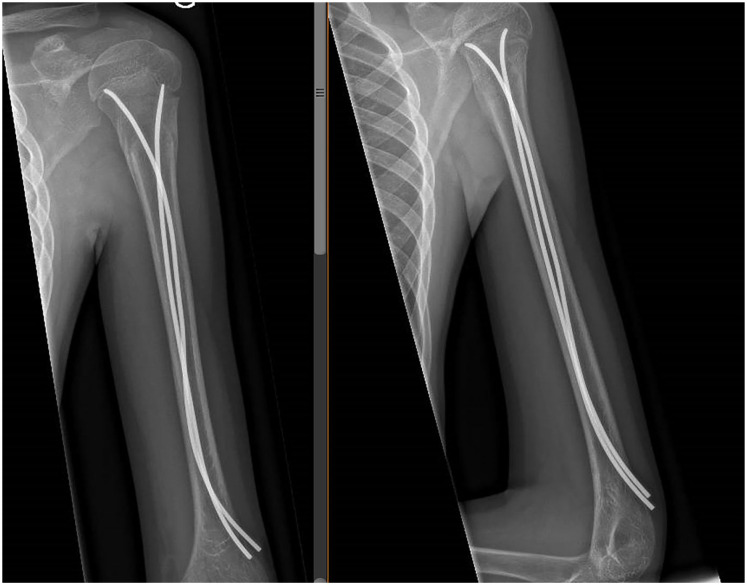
X-ray at 6-week follow-up with the DNT showing radiological union.
